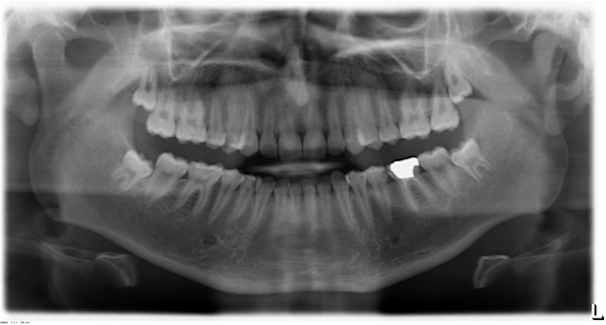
Orthopantomogram of the jaws and teeth. Lower third molars show incomplete root formation.
